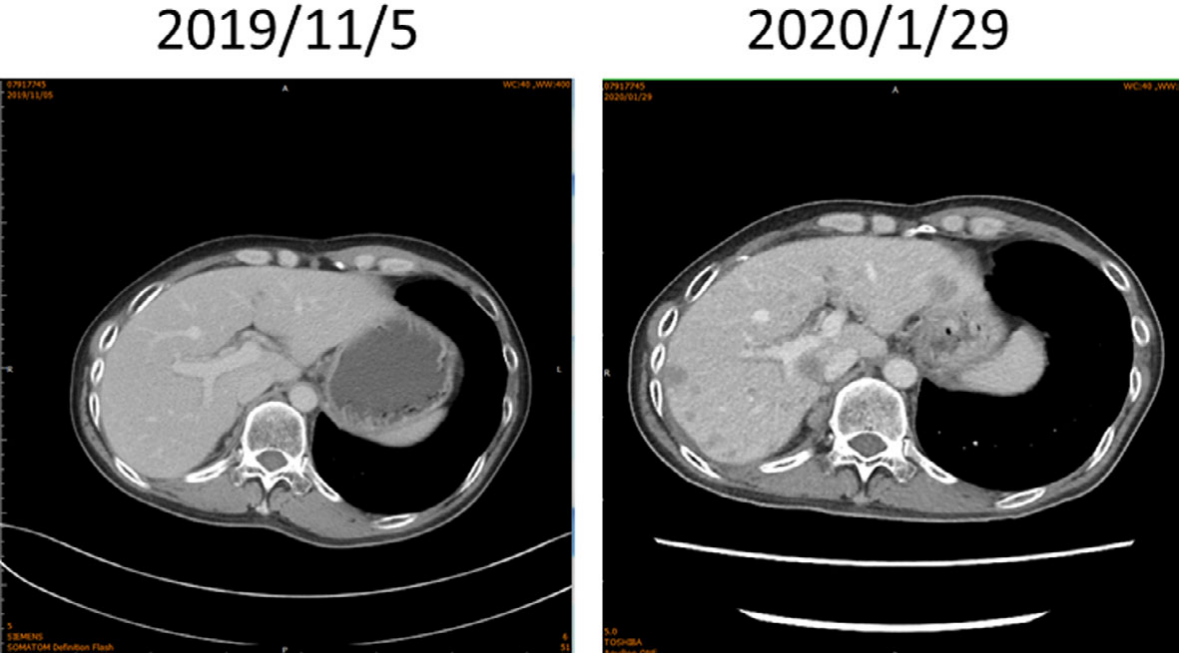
Serial CT scans showed liver metastasis progression during sorafenib treatment.
